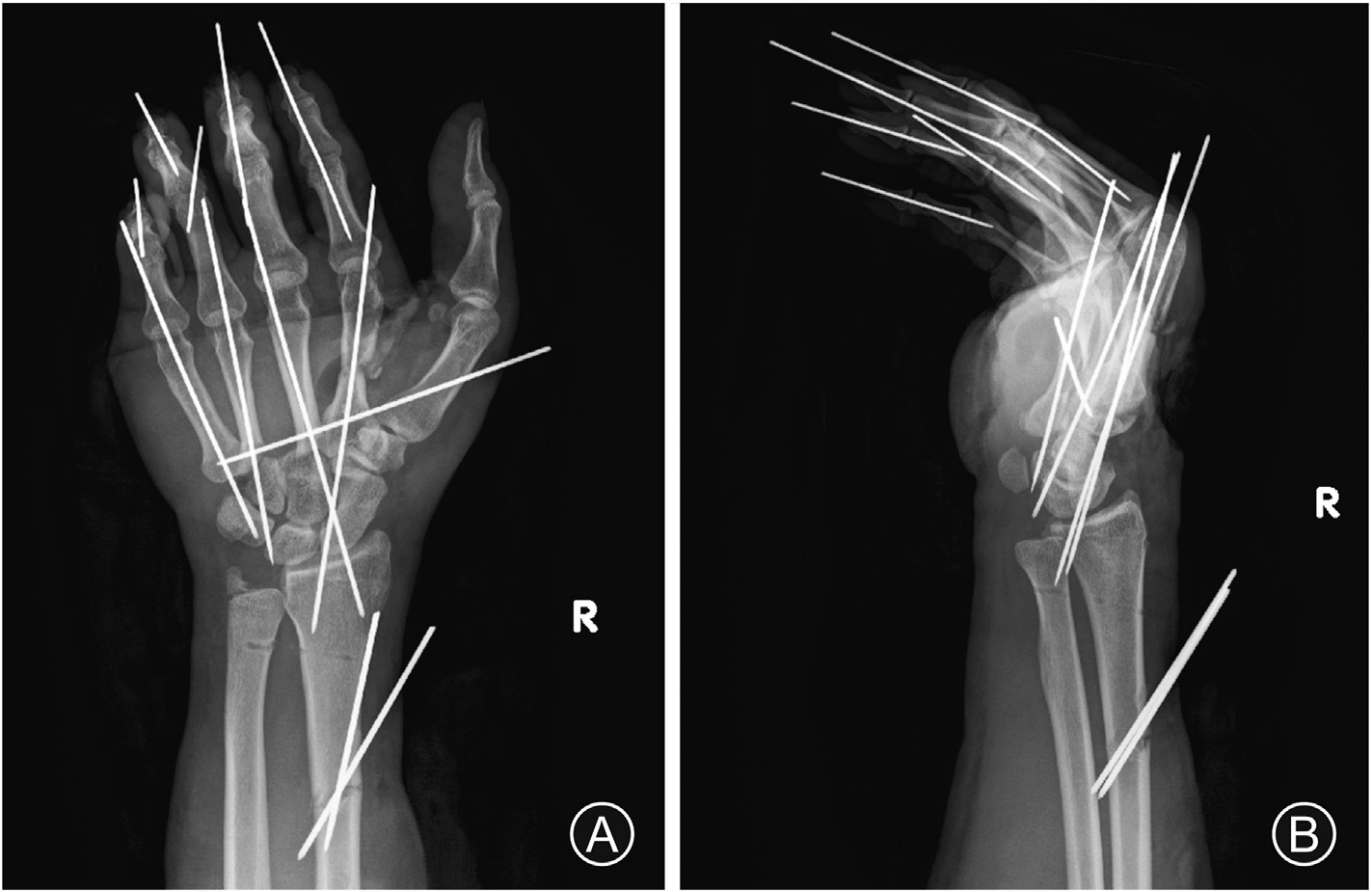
Radiographs showing the metacarpophalangeal joints fixed with block pins and the distal interphalangeal and passive proximal interphalangeal joints temporarily fixed with a K-wire (A: frontal view, B: lateral view).
